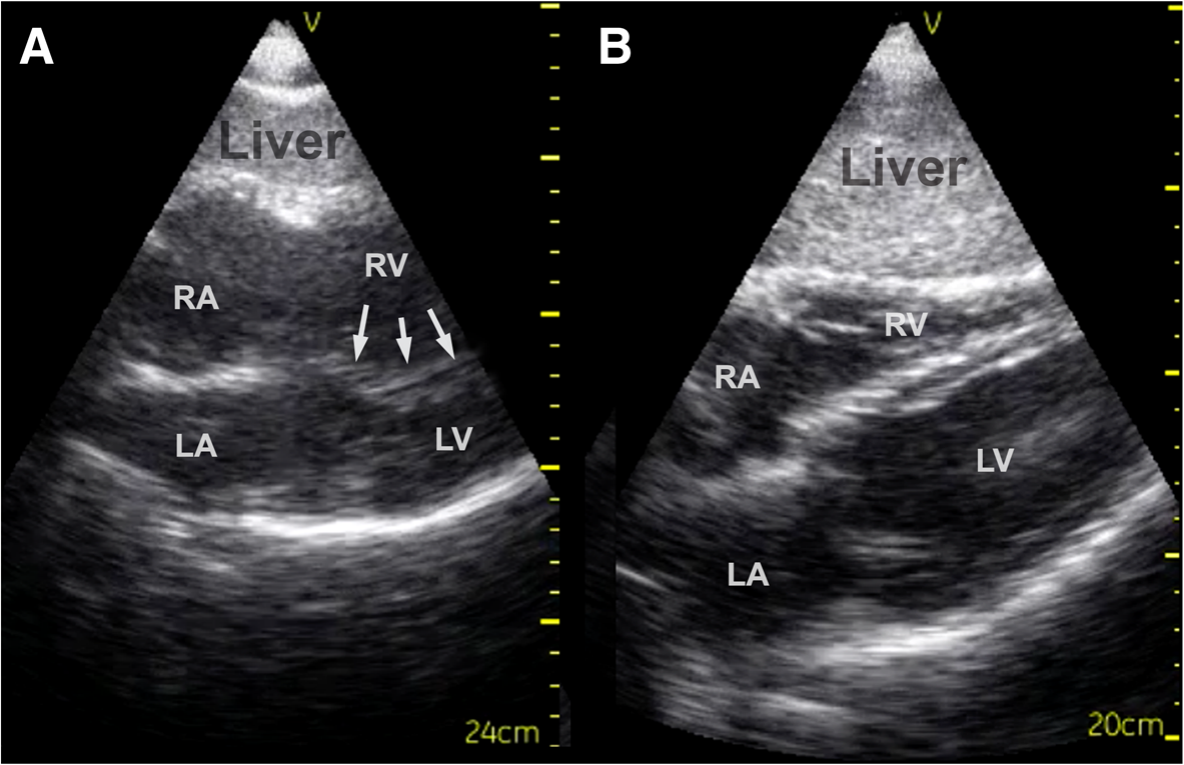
Subcostal 4-chamber view – pulmonary embolism and normal. A: Subcostal 4-chamber view - pulmonary embolism. Notice the dilated right ventricle and bulging of the septum into the left ventricle in the pathological echocardiogram because of the increased pressure in the right ventricle (arrows). The pattern of the septum can often be seen even when image-quality is low. B: Subcostal 4-chamber view – normal. RA = Right Atrium, RV = Right Ventricle, LA = Left Atrium, LV = Left Ventricle.
